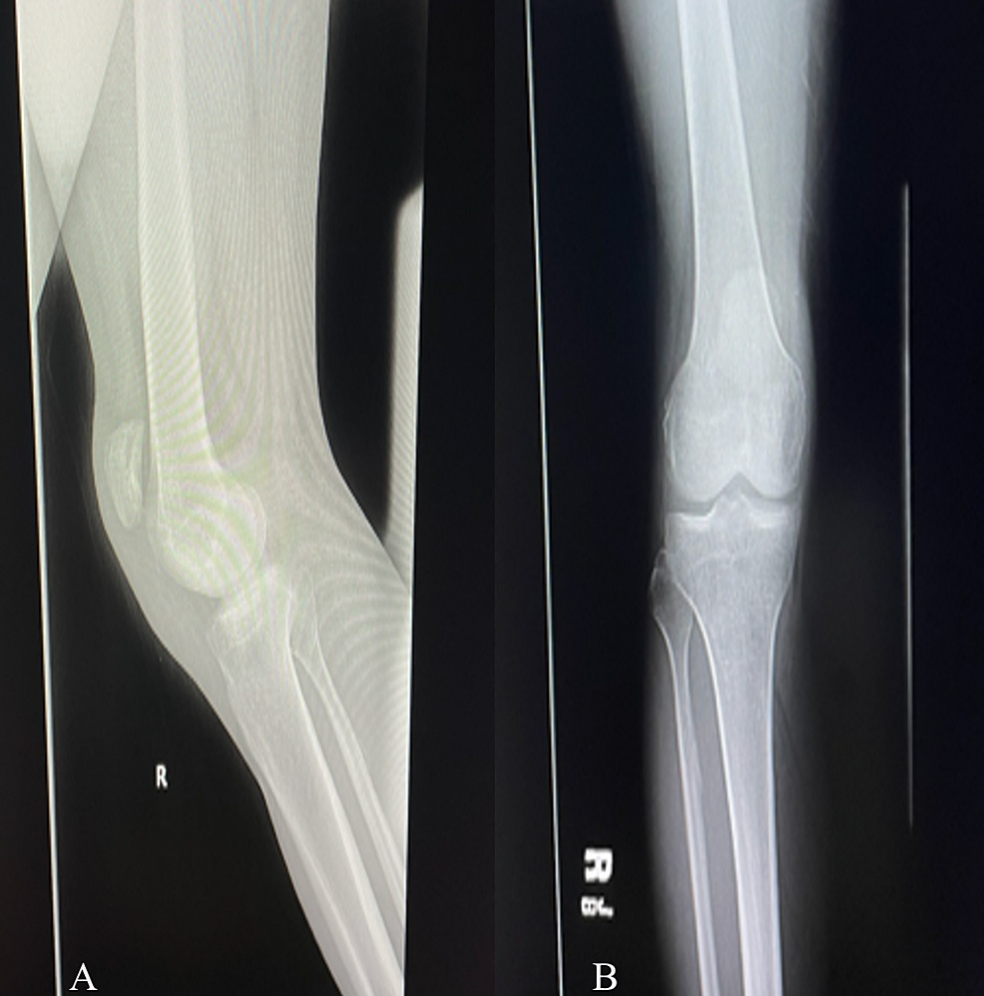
Radiograph of the right knee: (A) lateral view and (B) anteroposterior view showing the high-riding patella.
